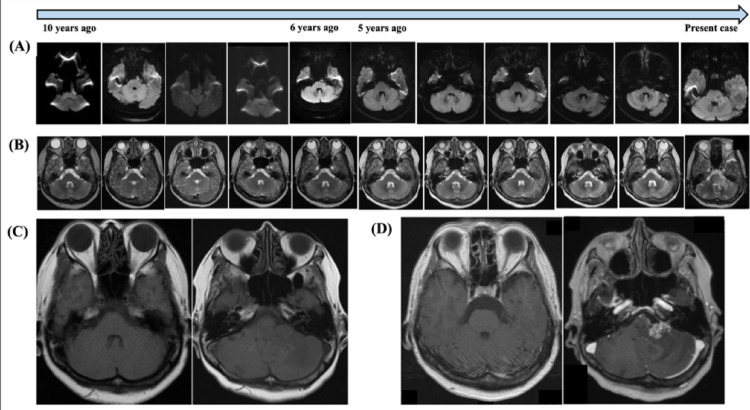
Serial imaging results, a, b) Serial images from diffusion-weighted imaging (DWI) (a) and T2-weighted imaging (T2WI) (b) on magnetic resonance imaging (MRI) during 10-year follow-up. Representative slices were selected to best demonstrate the lesion on each imaging study. c, d) Axial T1-weighted MRI at initial detection (c) and just before surgery 10 years later (d) (left panels: without Gd enhancement; right panels: with Gd enhancement)
